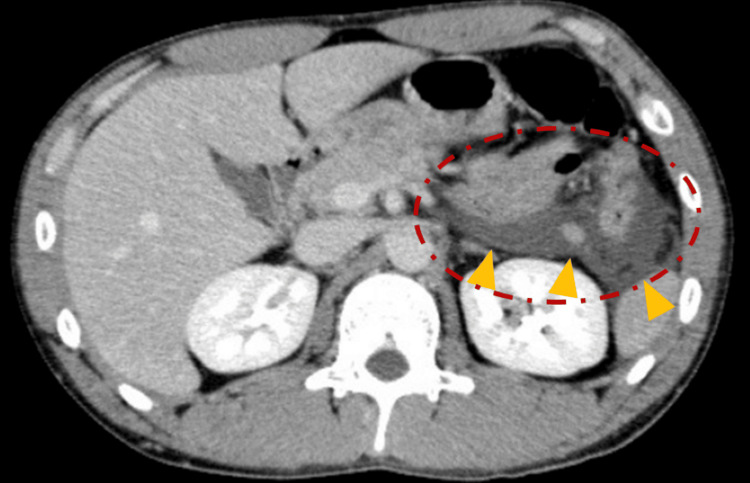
Contrast-enhanced CT revealing inflammation around the pancreas (red circle), extending into the left anterior pararenal space (yellow triangles).
